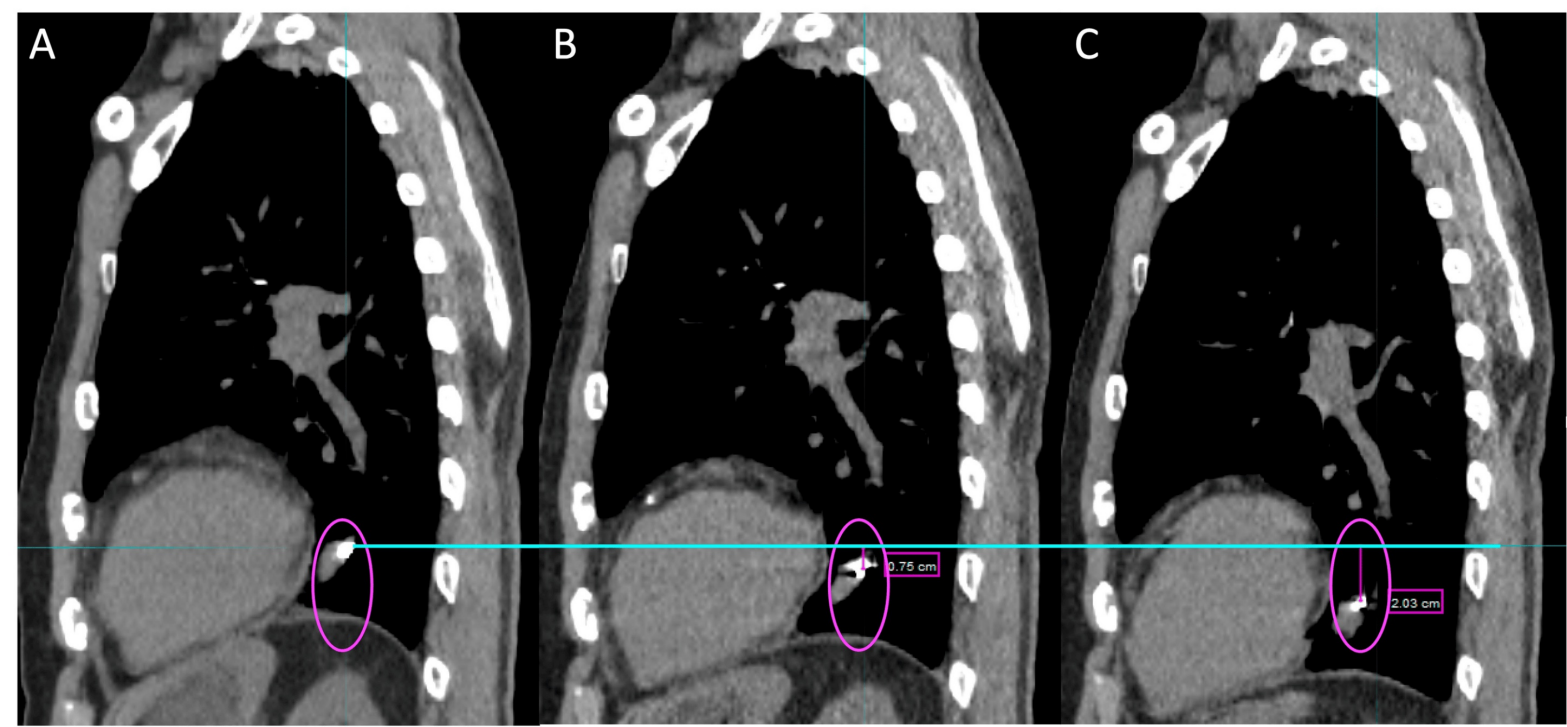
Example of sagittal images from the planning CT (A), exhale phase of the 4DCT (B), and inhale phase of the 4DCT (C), used to evaluate fiducial excursion. (A) Breath-hold exhale planning CT; (B) Exhale phase of 4DCT; (C) Inhale phase of 4DCT.
